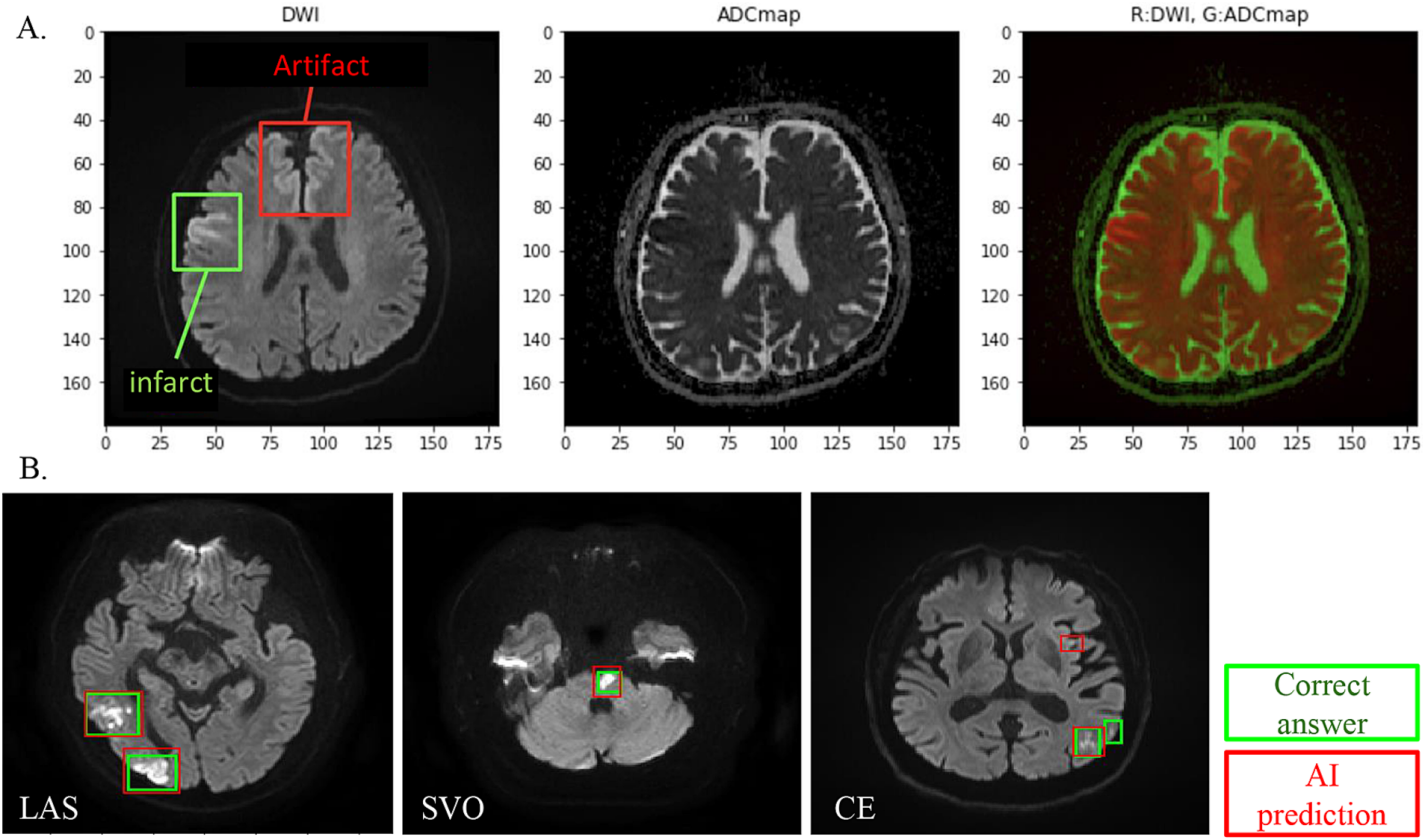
(A) DWI and ADC combined analysis method for infarct detection AI visualizes and evaluates the difference between DWI high-intensity area and ADC low-intensity area, except for symmetrical high-signal areas and areas where artifacts are likely to appear. (B) Infarct detection AI overview. One hundred cases were studied, and 18 cases were evaluated. In infarct lesion units, the sensitivity (recall) was 80% and the match rate (lesion-by-lesion evaluation) was 84%.
